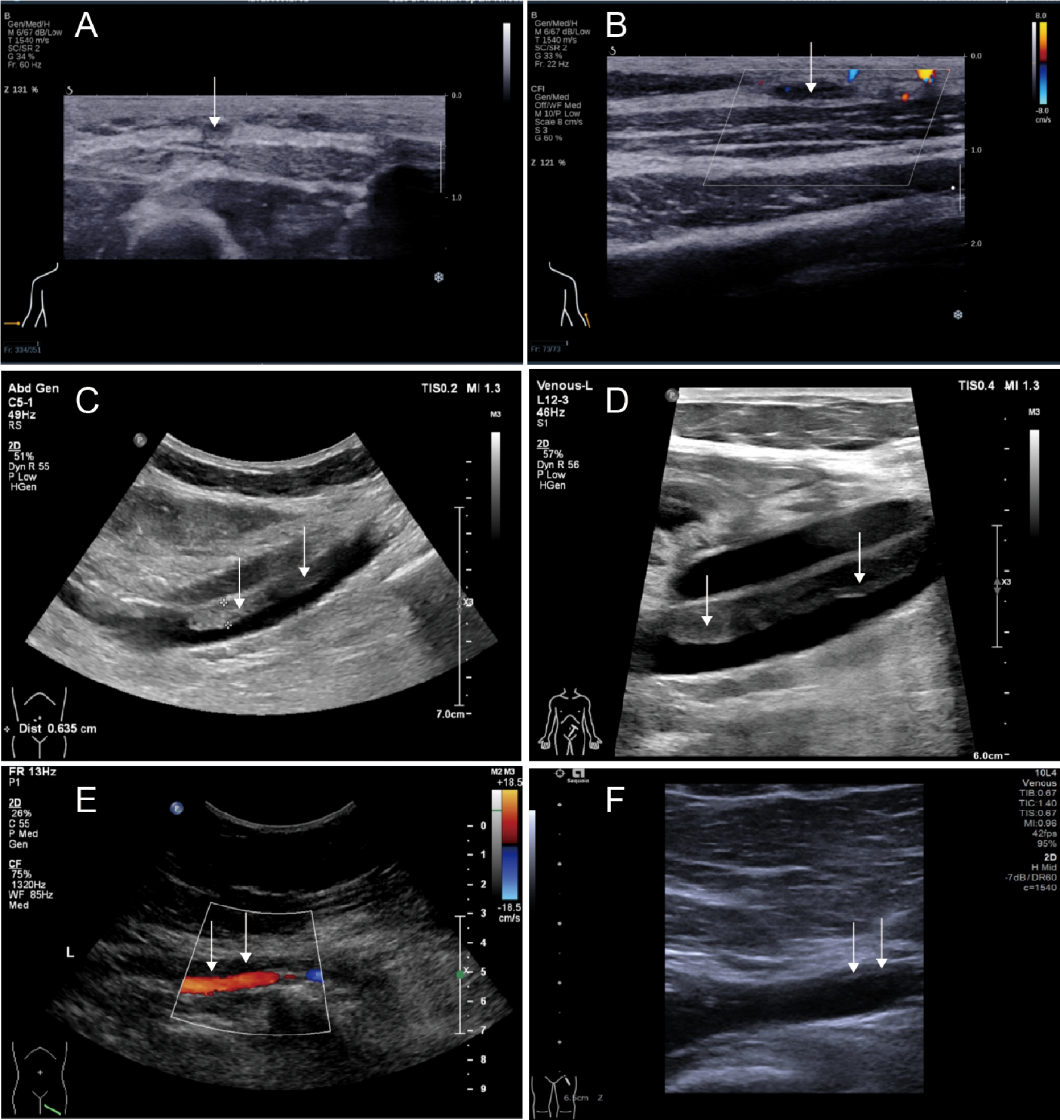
Ultrasonographic findings of multiple venous thromboses. (A) Right cephalic vein; (B) left cephalic vein; (C) right external iliac vein; (D) left external iliac vein; (E) left popliteal vein, (F) left common femoral vein.
